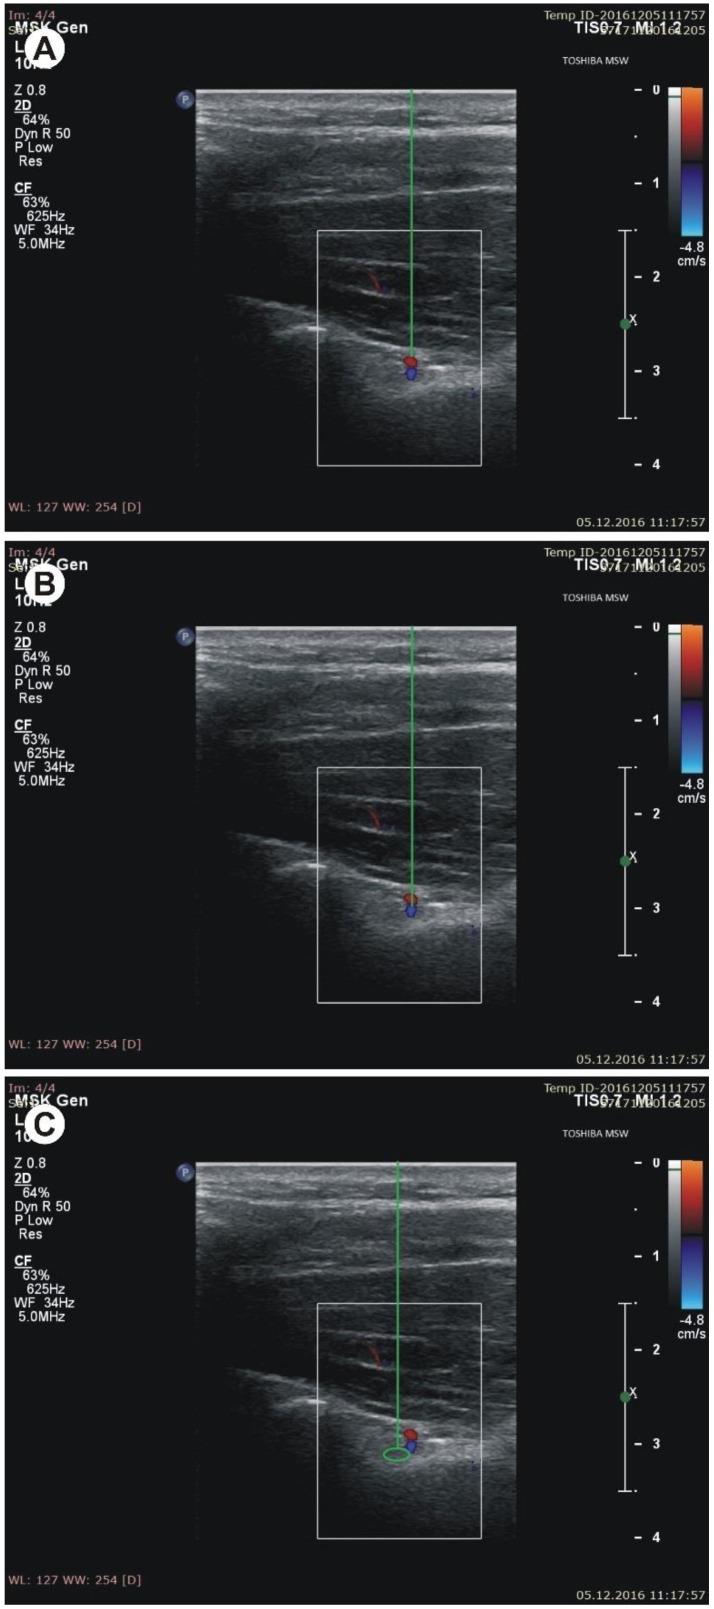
Measurements of the suprascapular notch region during ultrasonographic investigation. (A) the thickness of the soft tissue over the suprascapular artery; (B) the thickness of the soft tissue over the suprascapular vein; (C) the thickness of the soft tissue over the suprascapular nerve.
